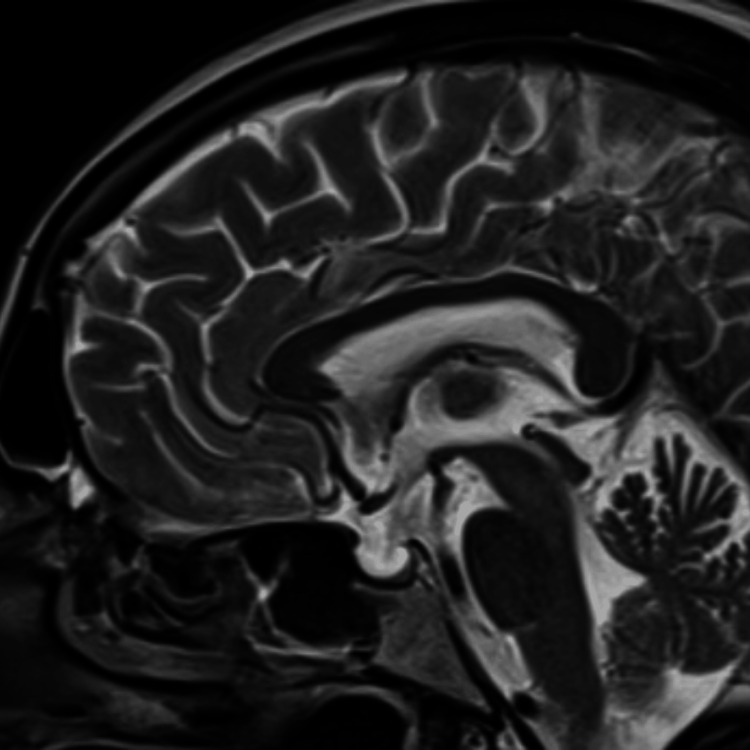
T2-weighted sagittal MRI image of the brain showing an empty sella filled with CSF CSF: cerebrospinal fluid
